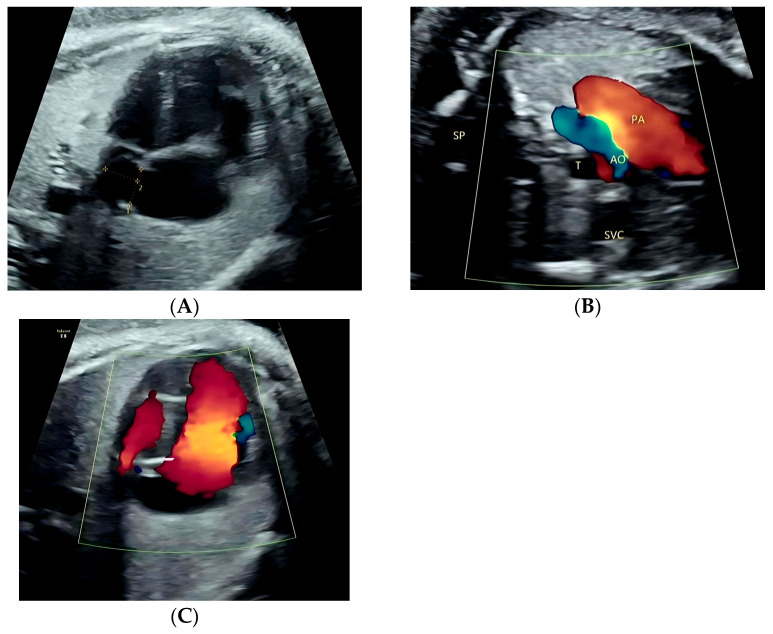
Prenatal ultrasound images from a false-positive case with a redundant foramen ovale flap. (A) At 35 + 4 weeks of gestation, the apical four-chamber view shows a redundant foramen ovale flap with mild atrial and ventricular asymmetry. (B) Great-vessel view showing disproportion between the pulmonary artery and the aorta. (C) Color Doppler apical four-chamber view more clearly demonstrating asymmetric color flow distribution between the right and left heart. Abbreviations: PA, pulmonary artery; AO, aorta; SVC, superior vena cava; SP, spine; T, trachea.
